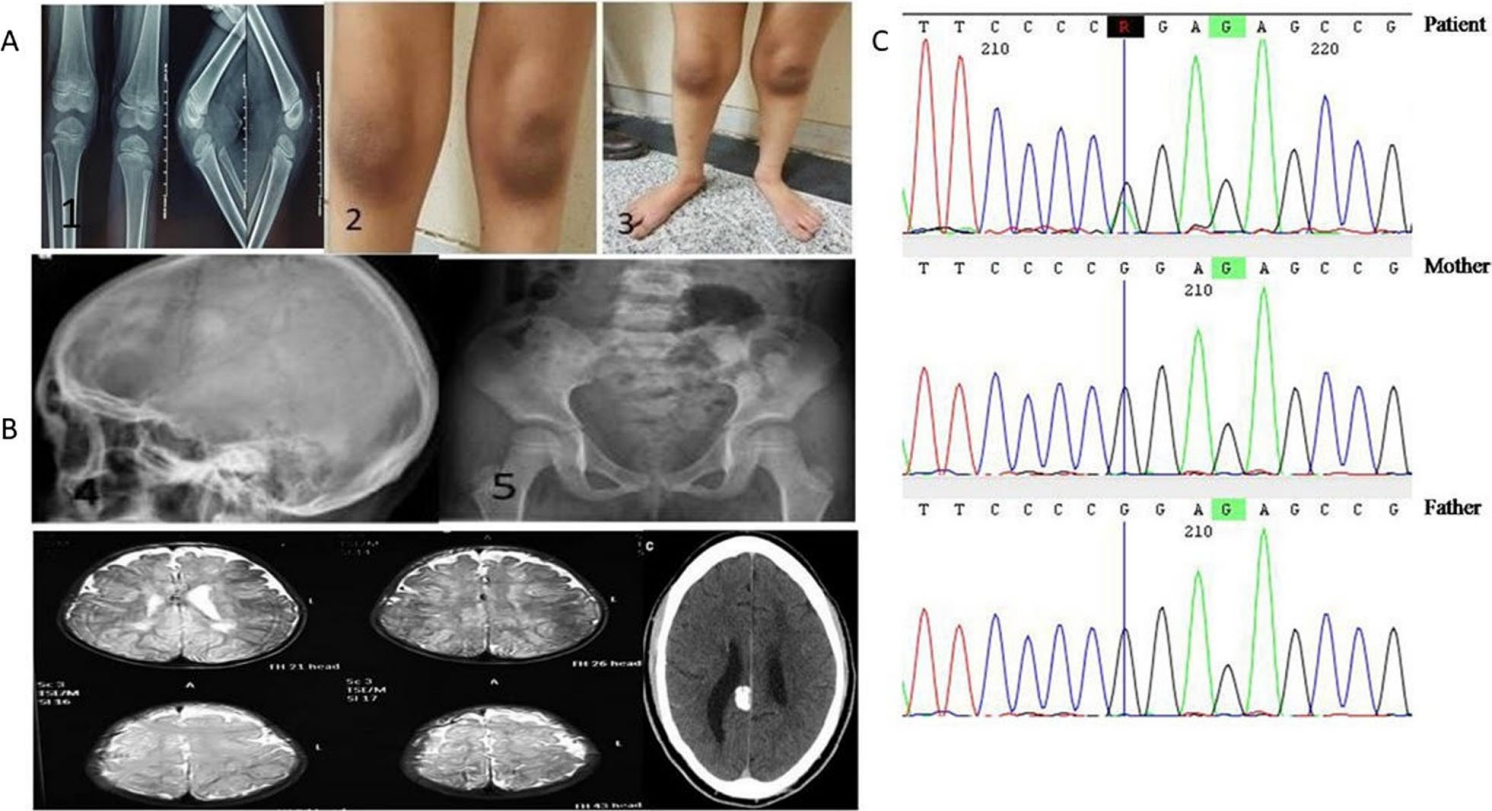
A Phenotypic characteristics of the patient; B Brain MRI of the patient. Imaging of the brain shows inappropriate myelination and disturbed white matter integrity; C Genetic test results. Sanger sequencing reveals a c.3147G>A; p.P1049P de novo heterogeneous mutation in this patient
